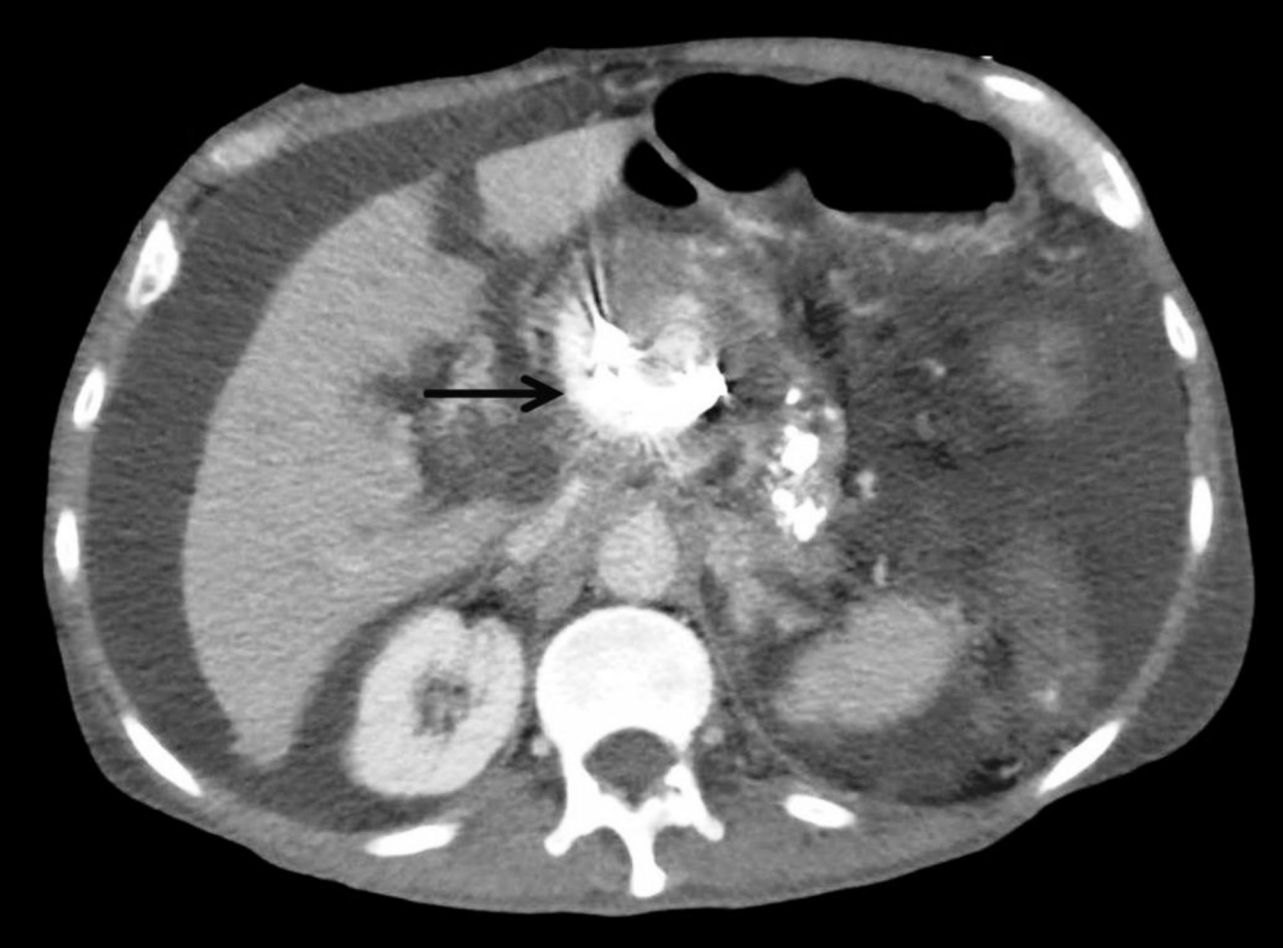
Computed tomography scan of the abdomen with pancreatic imaging protocol showing coil embolization (arrow) of the pseudoaneurysm in the head of the pancreas.
